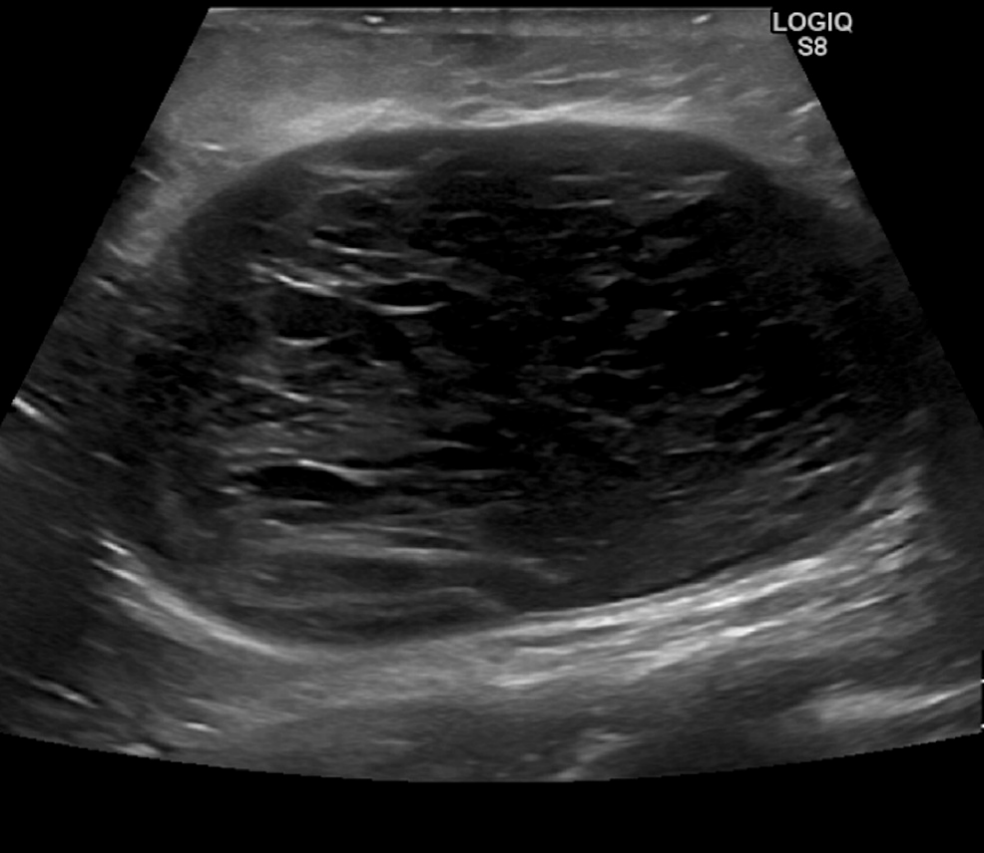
Breast Ultrasonography Image of a 15 x 10 x 5 cm breast seroma organized in pockets with hyperechoic capsule formation.
